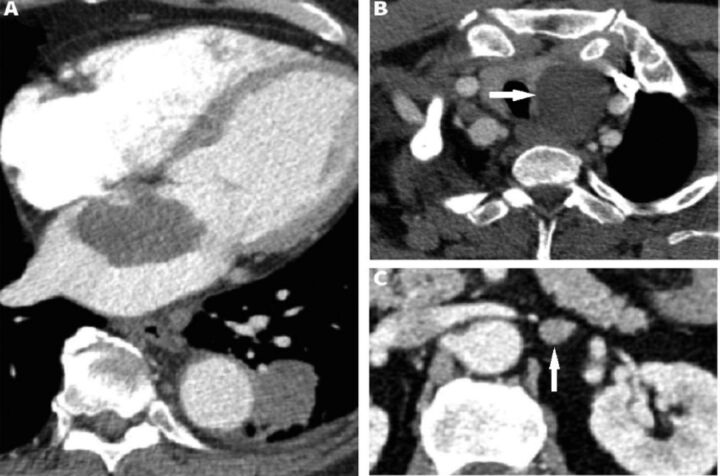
Enhanced computed tomography axial images demonstrating: A) 4.7 cm left atrial mass based on the interatrial septum at fossa ovalis typical for atrial myxoma, additionally, there is a left lower lobe mass; B) a large left thyroid lobe mass ; and C) a small left adrenal mass.
